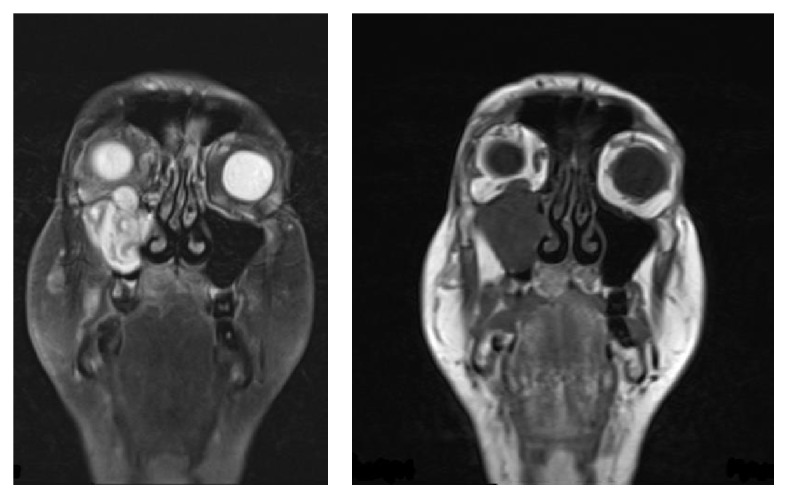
Postgadolinium MRI coronal sequence showing superior extension into the right orbit.
